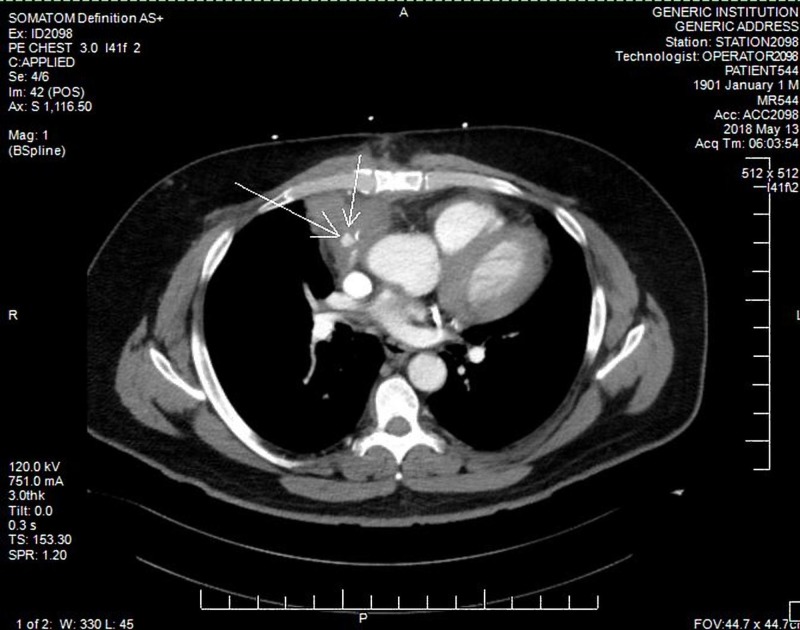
Computed tomography scan of the chest with contrast showing pseudoaneurysm in saphenous vein graft to right coronary artery, 2 cm from its origin, measuring 1.2 cm in size with adjacent fluid, possibly representing hemorrhagic debris.
